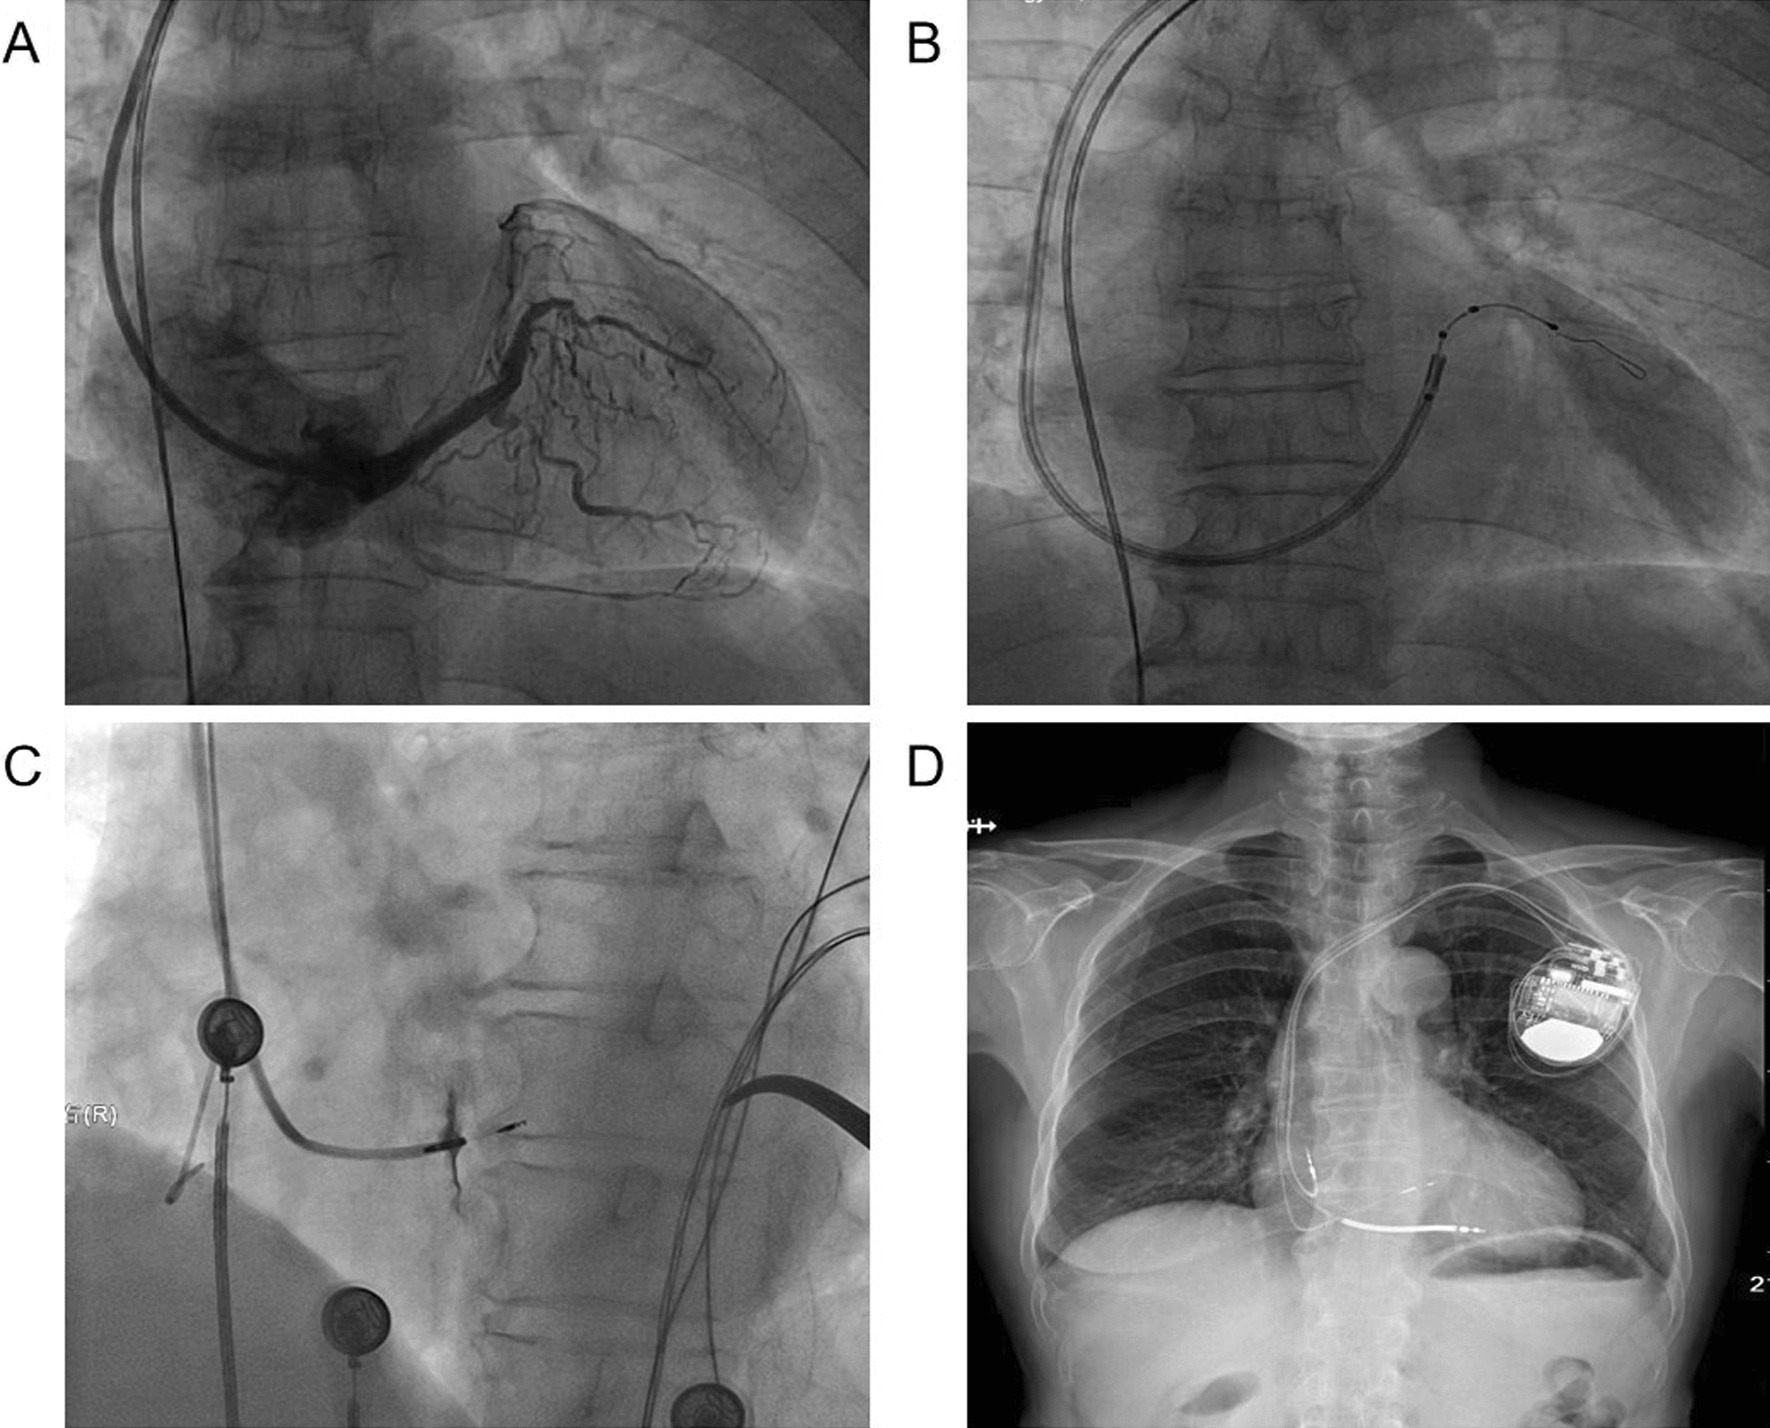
X-ray imaging of the interventional procedure process. A The coronary vein with severe distortion of the lateral posterior vein and a narrow and tortuous proximal posterior vein. B The left ventricular lead could not reach the target vessel. C Angiography of 3830 electrode in interventricular septum. D Final imaging after pacemaker implantation
